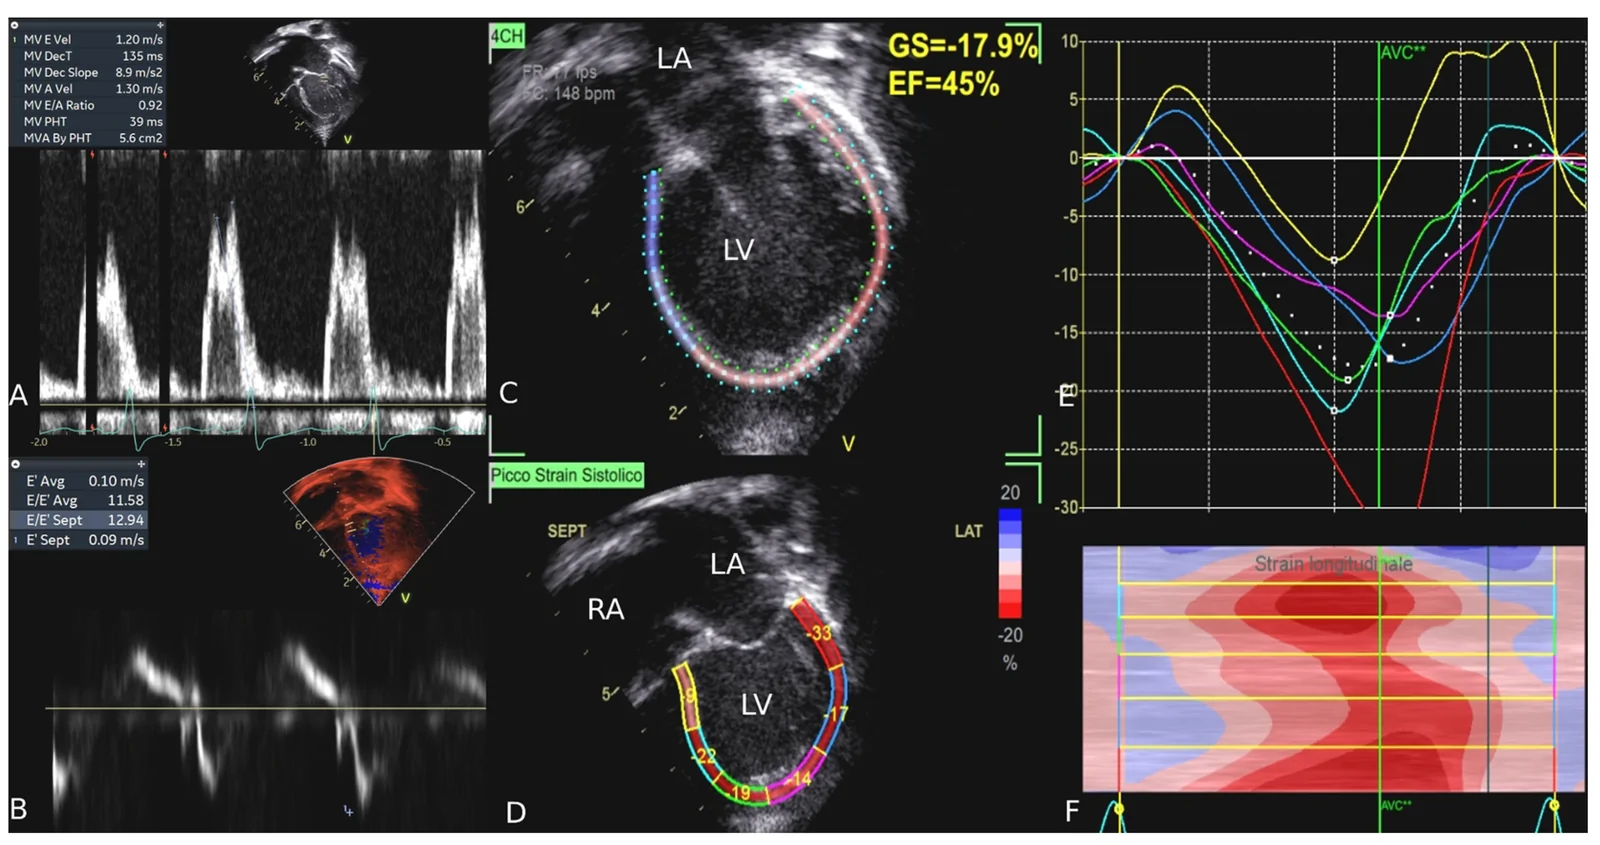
Comprehensive echocardiographic functional assessment during the acute heart failure presentation (Case 1). (A) Transmitral pulsed-wave Doppler showing an E/A ratio of 0.9. (B) Tissue Doppler imaging (TDI) demonstrating an E/E’ ratio of 12. (C) Apical four-chamber view with Speckle-Tracking Echocardiography (STE) overlay, highlighting the characteristic spherical, truncated left ventricular (LV) apex. (D–F) Longitudinal strain curves demonstrating mildly reduced global longitudinal strain (GLS = −17.9%) and an ejection fraction of 45%. The strain analysis reveals a regional base-to-apex functional gradient. RV = Right Ventricle; LA = Left Atrium.
